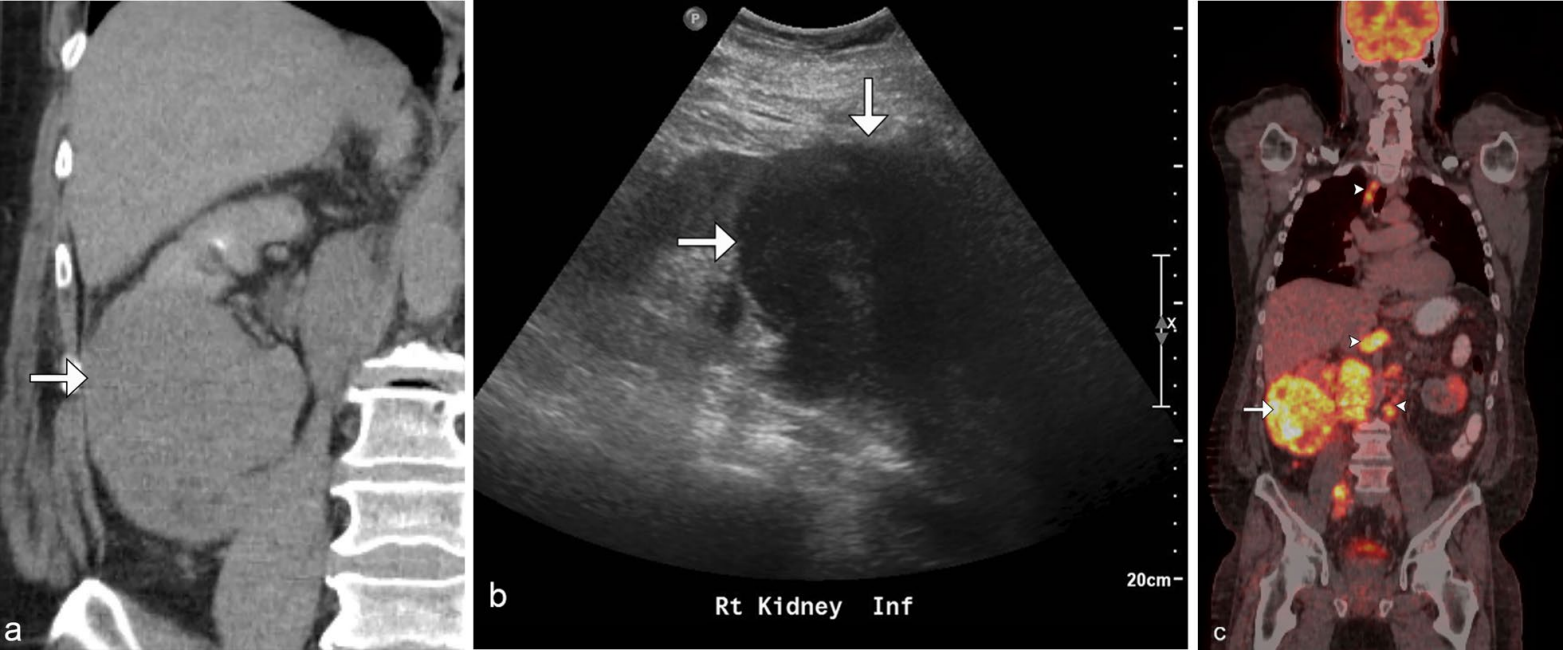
Solitary mass of renal lymphoma.* Coronal CT delayed post contrast image (a) demonstrates a hypoenhancing right lower pole renal mass with hypoechoic appearance on ultrasound (b) and marked F18-FDG avidity on PET/CT (c) (arrows). Additional FDG avid supradiaphragmatic and extensive infradiaphragmatic lymphadenopathy is helpful in confirming the diagnosis of lymphoma (arrowheads)
